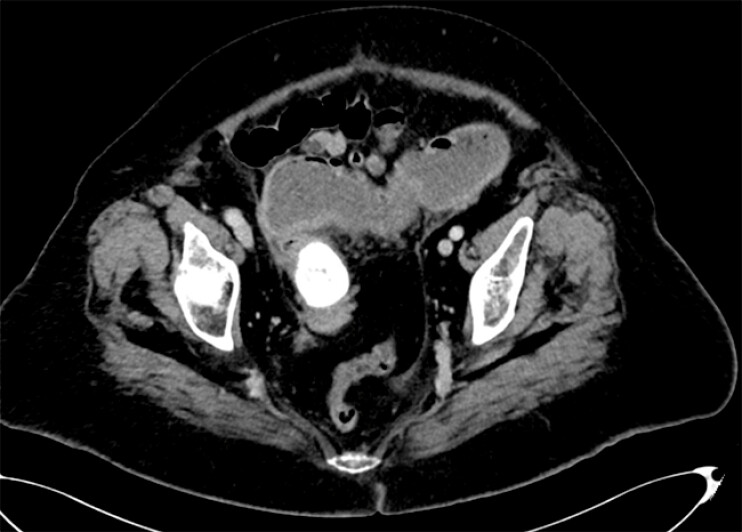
Abdominal CT revealing sigmoid obstruction caused by a 40-mm calcified intraluminal mass. CT, computed tomography.
